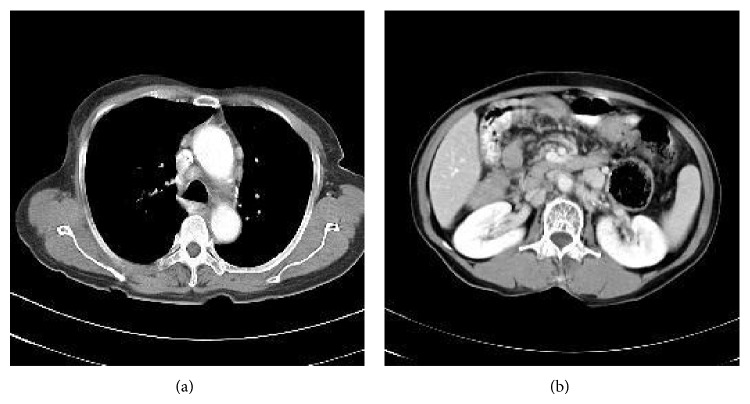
(a) A follow-up chest CT after 3 cycles of ABVD chemotherapy shows dramatic improvement of the lymphadenopathy in the mediastinum. (b) As compared with previous APCT, enlarged lymph nodes are decreased in number and size after 3 cycles of ABVD chemotherapy.
